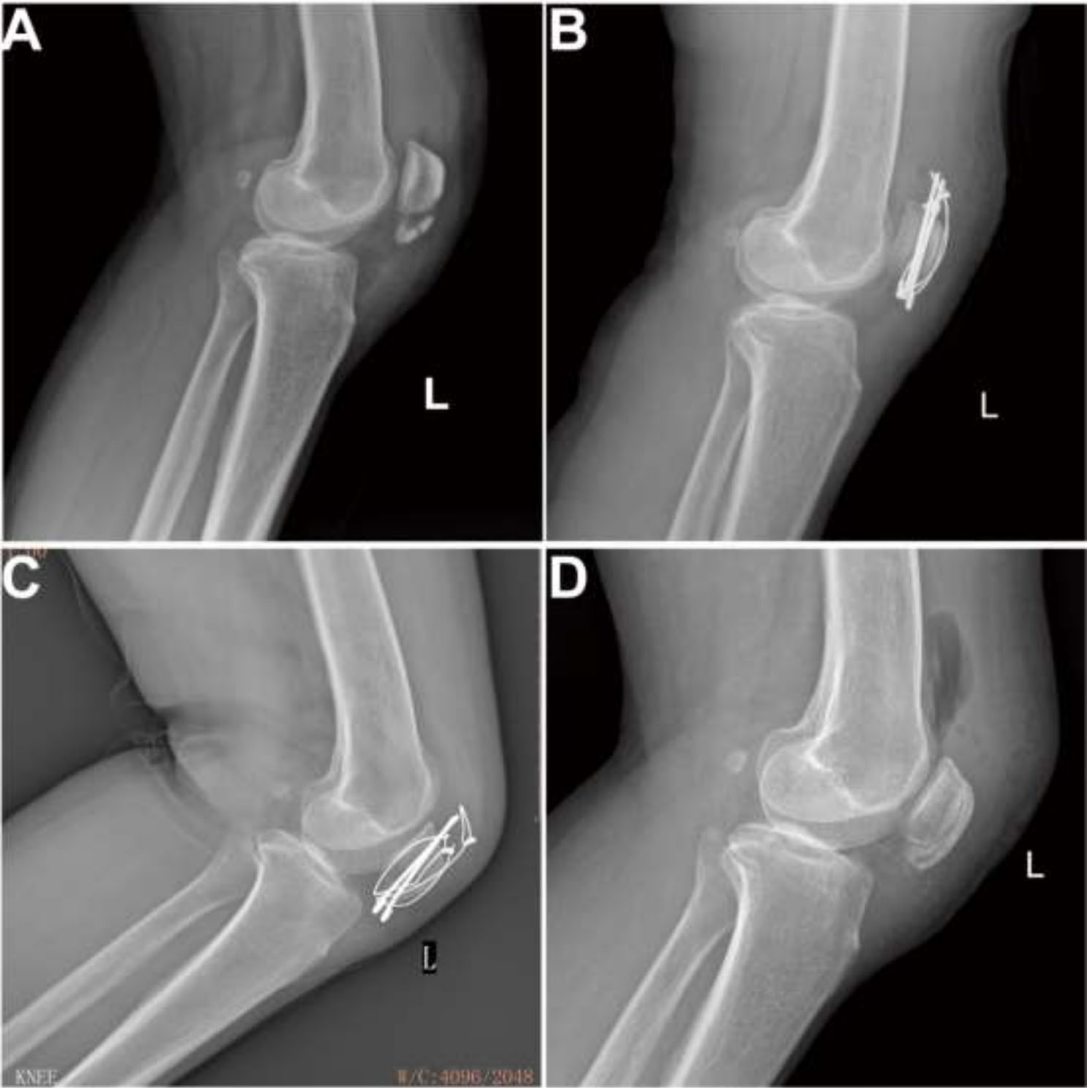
Case vignette No 2. A 52-year-old man with a comminuted inferior pole patella fracture (left) from a fall injury with an AO classification of 34-A1 underwent a Kirschner-wire tension band combined with patellar cerclage technique. (A) Preoperative radiographic results. (B) Fracture fixed by the Kirschner-wire tension band combined with patellar cerclage technique. (C) X-Ray presentations of the broken wire at 13 months after surgery. (D) X-Ray image after the implants were removed
